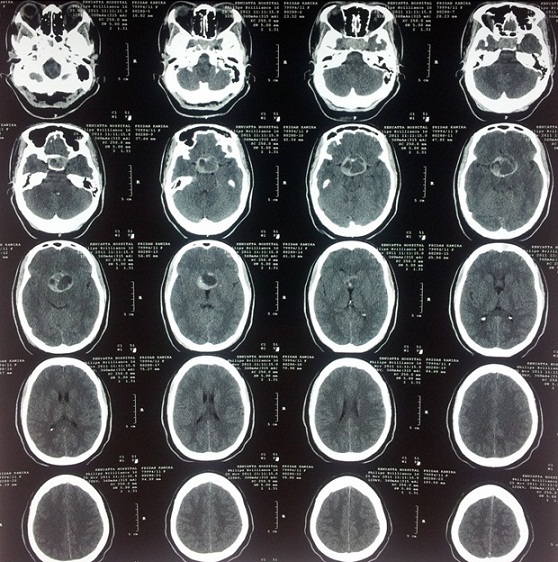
CT scan with contrast. Axial slices
